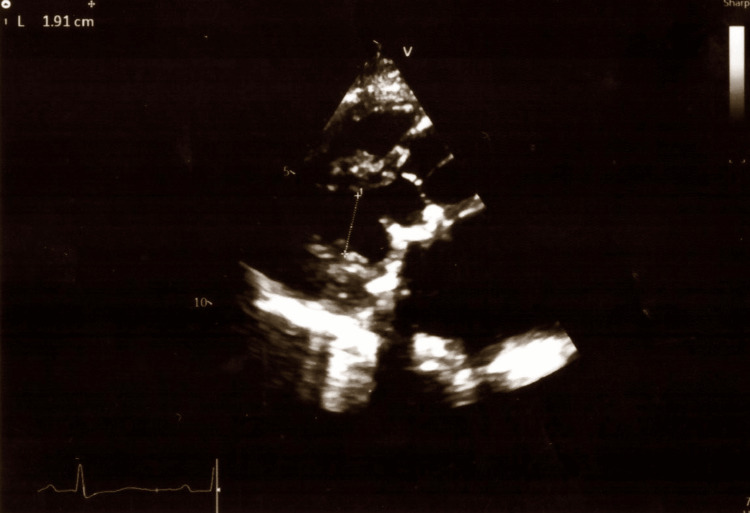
Parasternal long-axis echocardiogram of the patient with mitral valve stenosis
